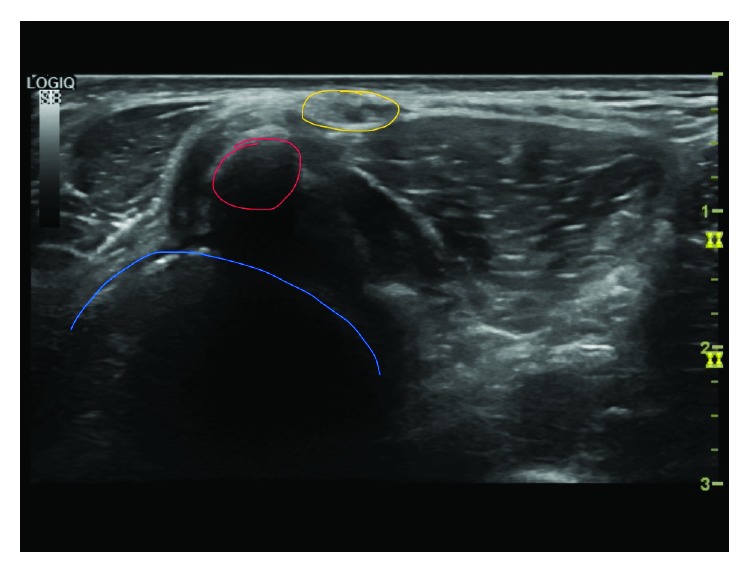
Axial ultrasound of the posterior lateral knee (blue: femoral condyle, red: fabella causing acoustic shadowing, and gold: peroneal nerve).
